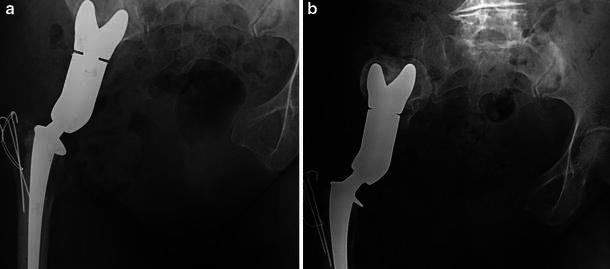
X-ray films of patient P.L., 68 y.o. at the time of operation.a Postoperative AP view of the pelvis; the saddle is seated through a notch in the iliac wing.b Same view 38 months afterwards. The patient reported suffering from pain during weight bearing for the previous 2 years. Note the proximal migration of the saddle in relation to the wearing of the iliac wing
